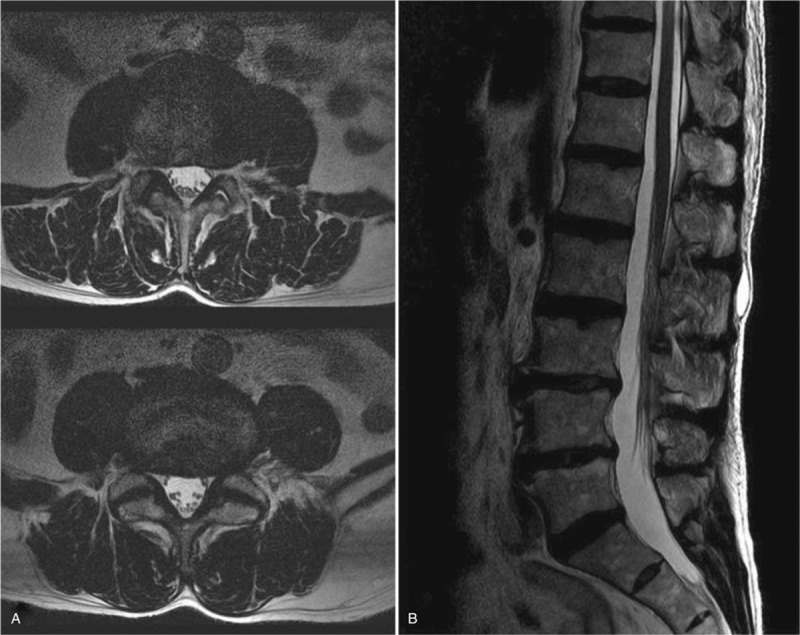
Axial (A) and sagittal (B) views of T2-weighted magnetic resonance images of the lumbar spine show mild disc protrusions of L3-4 and L4-5.
